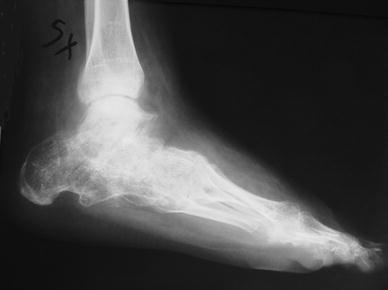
Post-operative plain X-ray of the foot after frame removal (lateral view)
